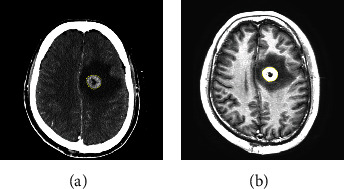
Typical contours of brain metastases on CT and MRI images.
